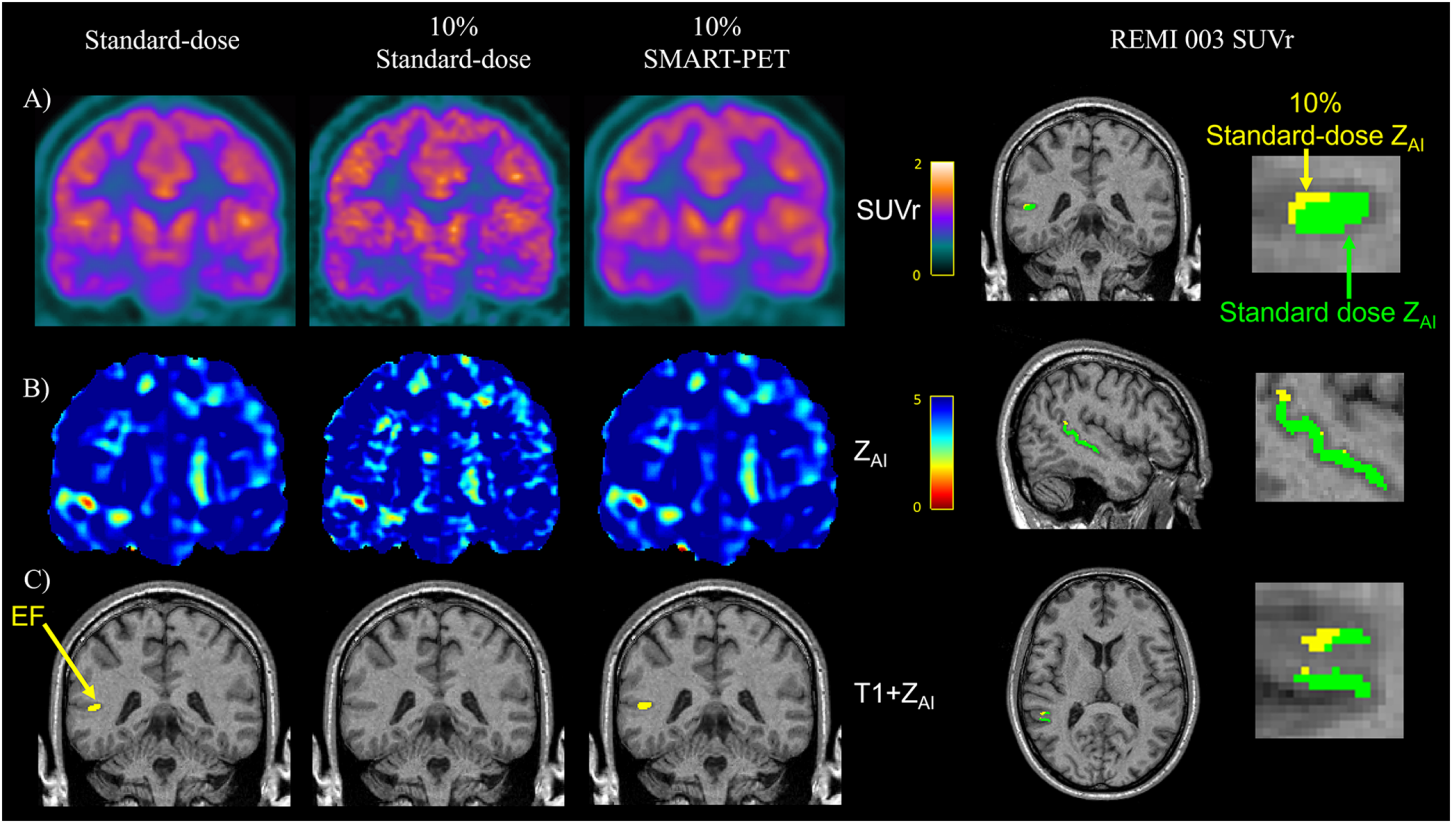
Visual assessment in an epilepsy case reveals similar (A) PET SUVr map, (B) Z-score map generated from AI mapping (Z_AI_ map), and (C) hypometabolic PET ROI (yellow) between standard-dose and SMART-PET denoised PET images. *EF, epilepsy focus.* The images are coronal brain slices of an adult drug-resistant epilepsy patient [52-year-old male, patient#3 in (15)].
